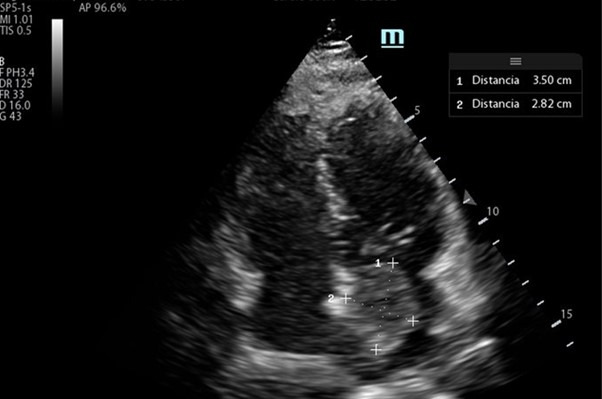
Apical 4 chamber view. Mass in left atrium.
